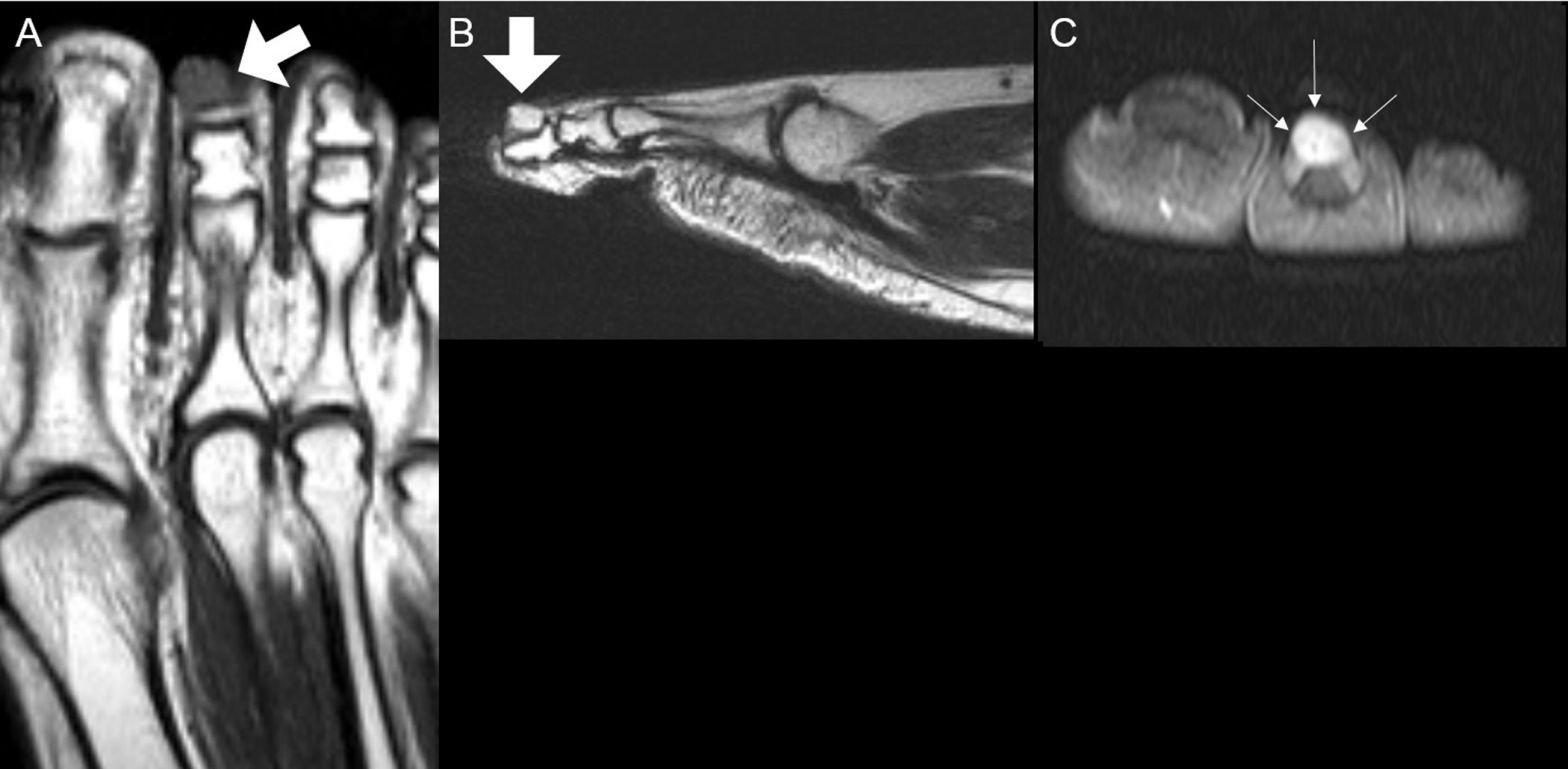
A 31-year-old woman with digital schwannoma in the subungual area of the 2^nd^ toe. (A) On coronal T1-weighted image, the mass demonstrates mild hyperintense SI compared to skeletal muscle (arrow). (B) On sagittal T2-weighted image, the mass reveals homogeneously hyperintense SI compared to skeletal muscle (arrow). (C) On axial contrast-enhanced T1-weighted image, the mass demonstrates homogeneous enhancement with displacement of the nail plate (thin arrows). *SI*, signal intensity.
